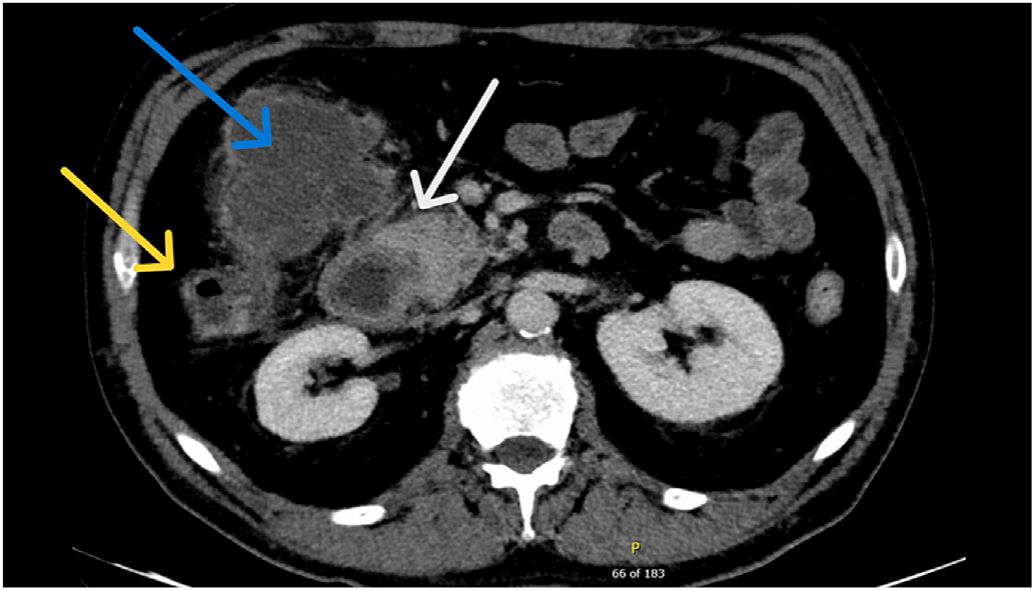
Axial CT angiogram of the abdomen in the arterial phase demonstrating large rim-enhancing fluid collection (blue arrow) in the right upper quadrant consistent with abscess. This collection abuts both the colon (yellow arrow) and duodenum (white arrow). There is no visualized site of bowel perforation, although this is not excluded.
